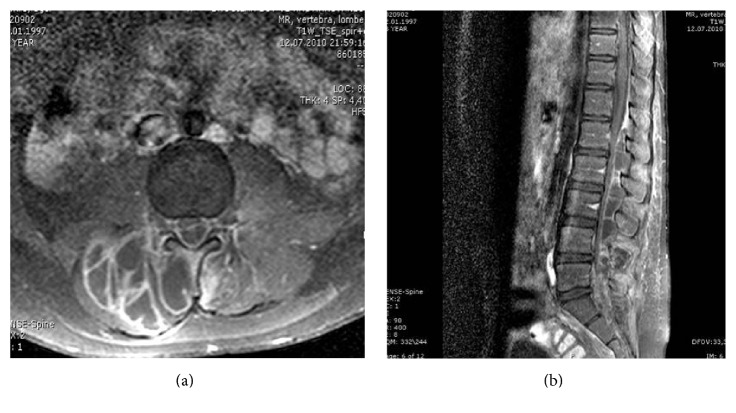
(a) Preoperative axial lumbar MRI. (b) Preoperative sagittal MRI.
